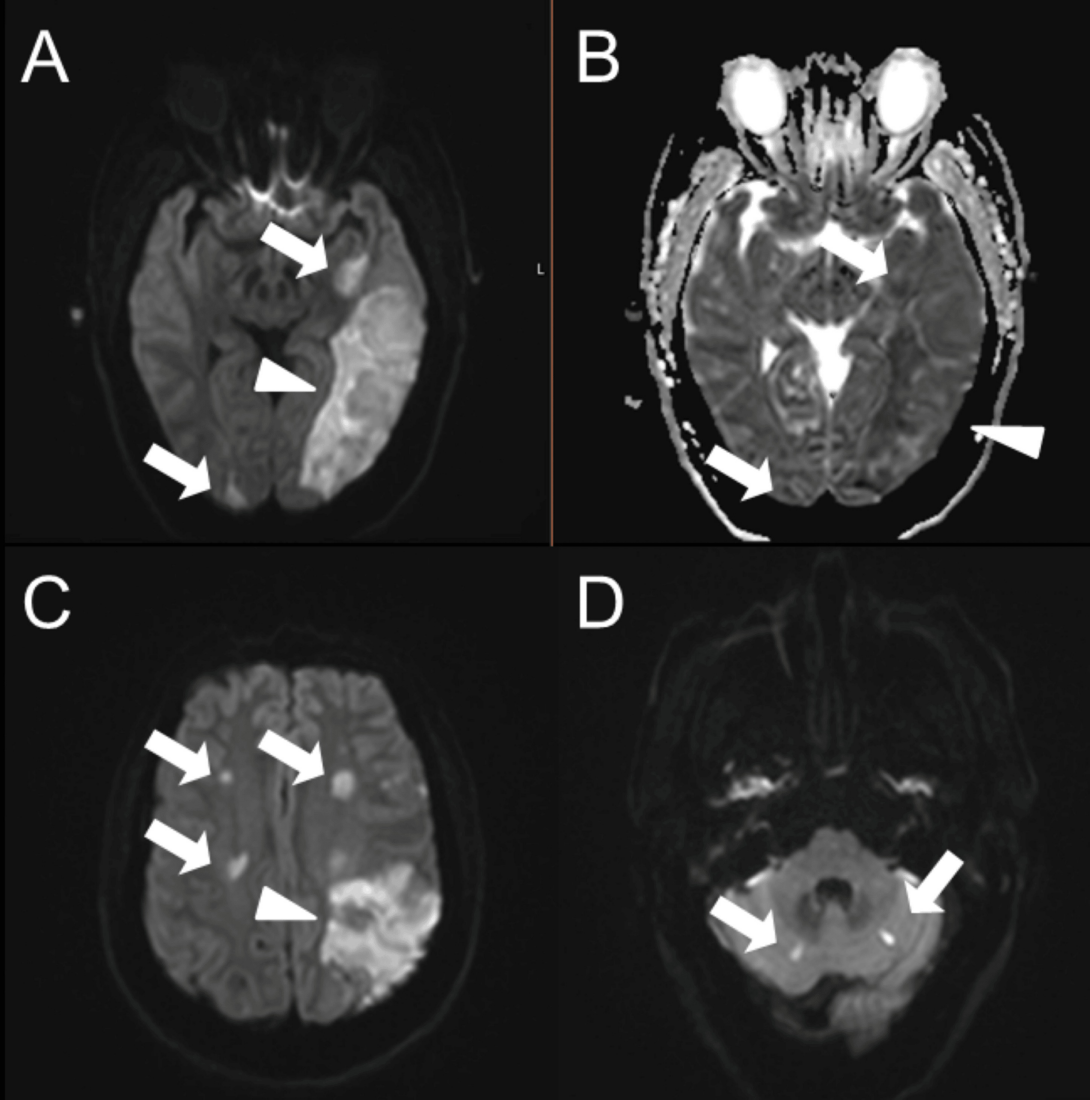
Follow-up brain MRI demonstrated progression to a large territorial infarct. Axial diffusion-weighted imaging (A) shows focal diffusion restriction in the left middle cerebral artery (inferior division) territory (arrowhead), along with multifocal infarcts (arrows), confirmed by true restricted diffusion on the corresponding ADC map (B). Additional DWI images (C and D) reveal small infarcts (arrows) in both hemispheres, involving the frontal, parietal, occipital, and temporal lobes, with associated mild mass effect. ADC: Apparent diffusion coefficient, DWI: Diffusion-weighted imaging
